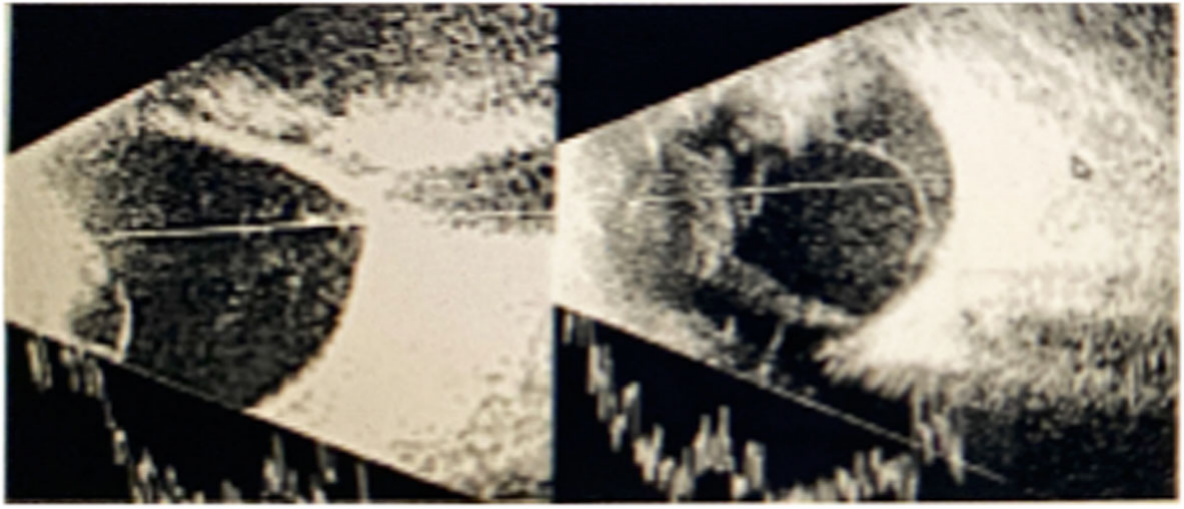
B-scan ocular ultrasonography shows medium to highly reflective floaters and membranous echoes with loculated opacities in the vitreous cavity more condensed posteriorly with choroidal thickening
